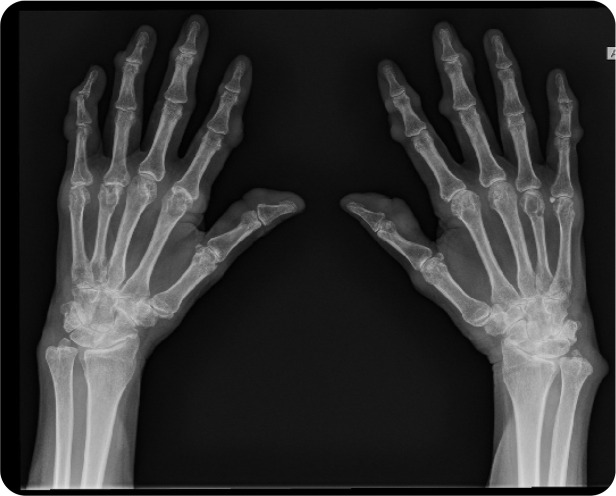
Hand and wrist x-rays of the same patient. Asymmetrical soft tissue swelling is evident in almost all digits, reflecting the presence of subcutaneous nodules. Severe destructive changes affecting all metacarpophalangeal joints and joint space narrowing are depicted bilaterally. Joint space narrowing and cyst formation cited in carpal bones. Finally, erosive changes are present in the right hand affecting the 3rd and the 5th distal interphalangeal joints.
